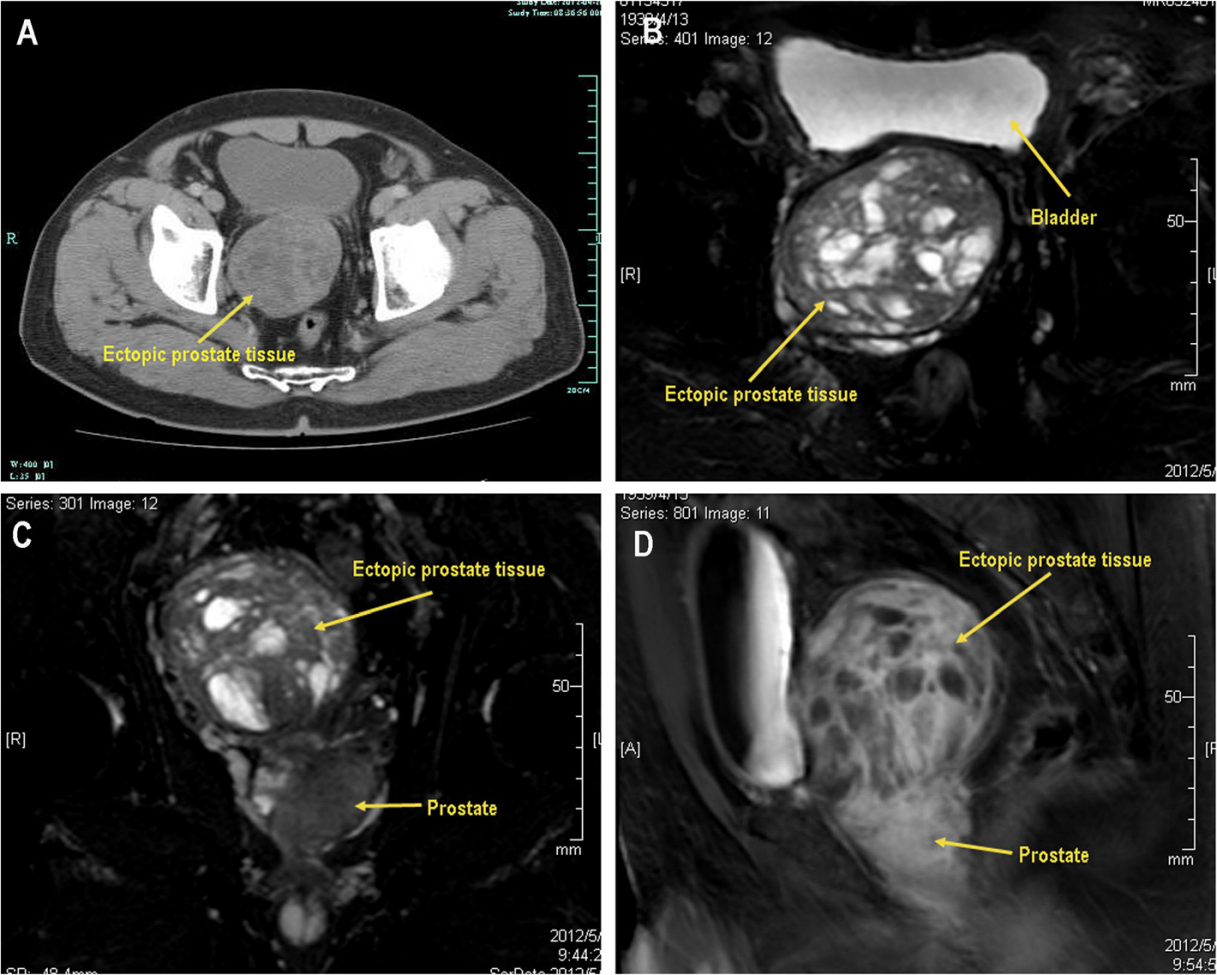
Pelvic computed tomography (A) demonstrated a heterogeneous contrast-enhanced mass (*arrow*) 8 cm in diameter in the retrovesical space. B, C, D: Pelvic magnetic resonance imaging showed a tumor (*arrow*) of heterogeneous intensity with a multilocular cystic structure.
